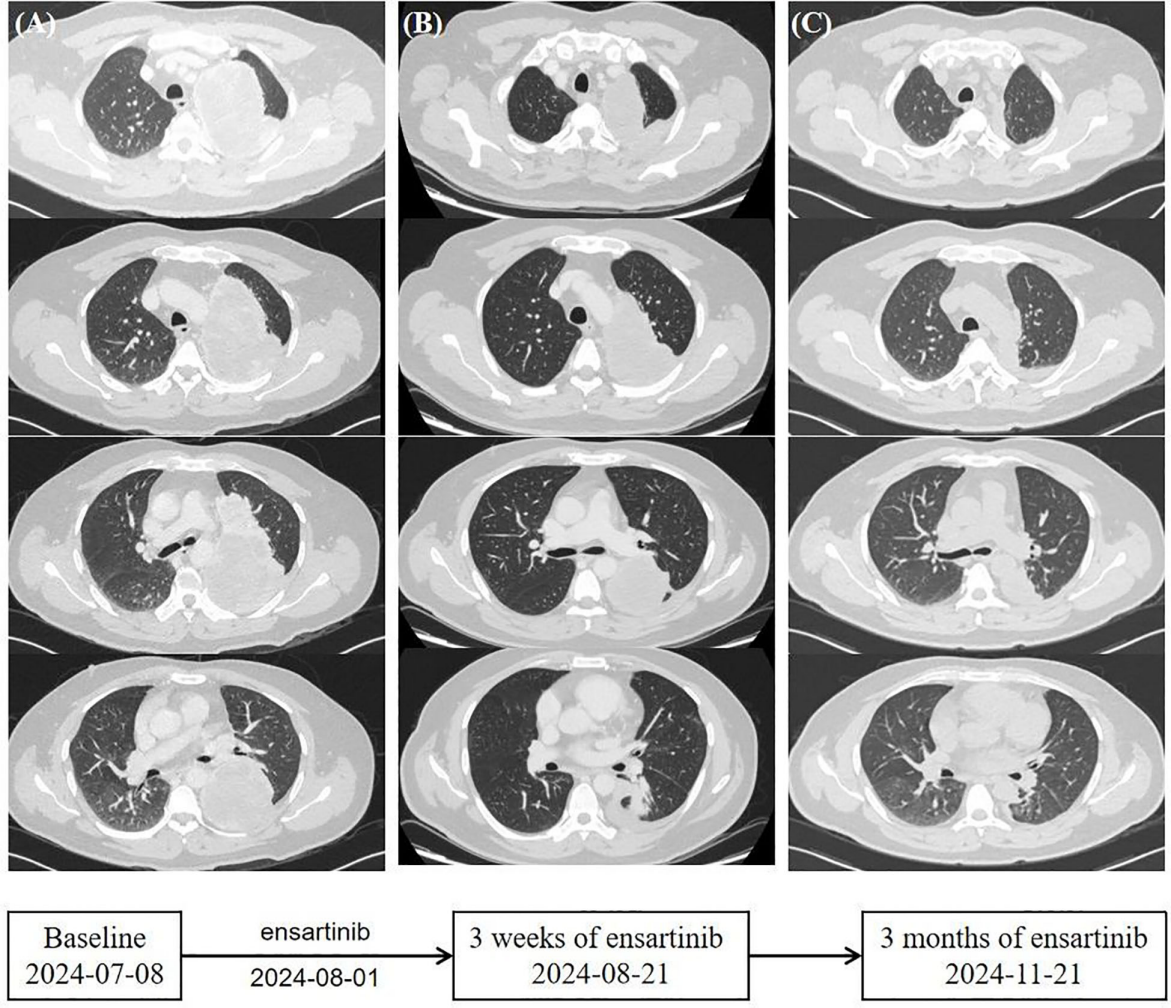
Timeline. (A) Computed tomography scans of the primary lung lesions at baseline before the initiation of ensartinib. (B) Ensartinib treatment for 3 weeks. (C) Ensartinib treatment for 3 months.
